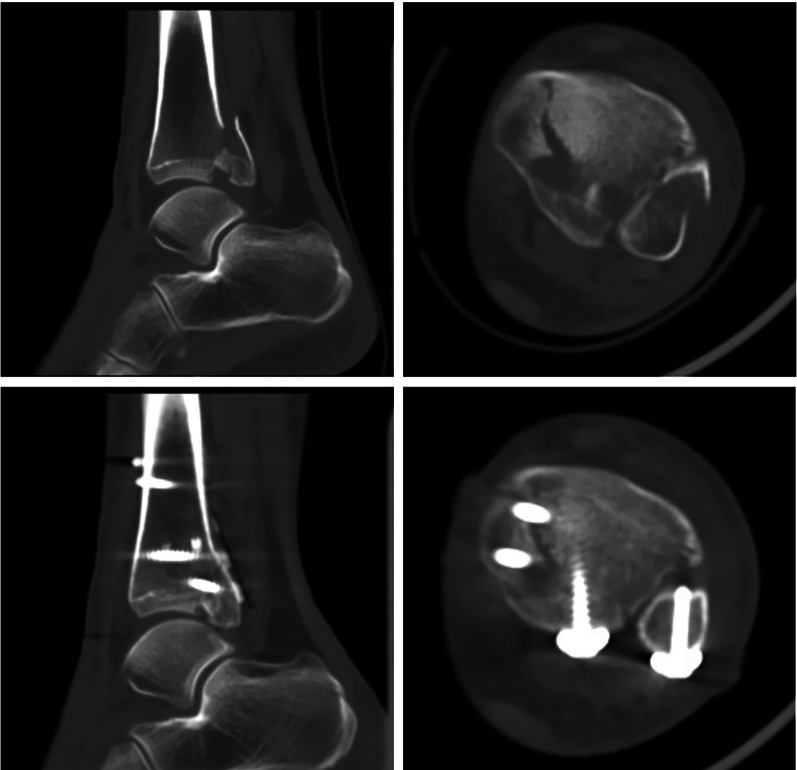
Pre- and postoperative CT images
